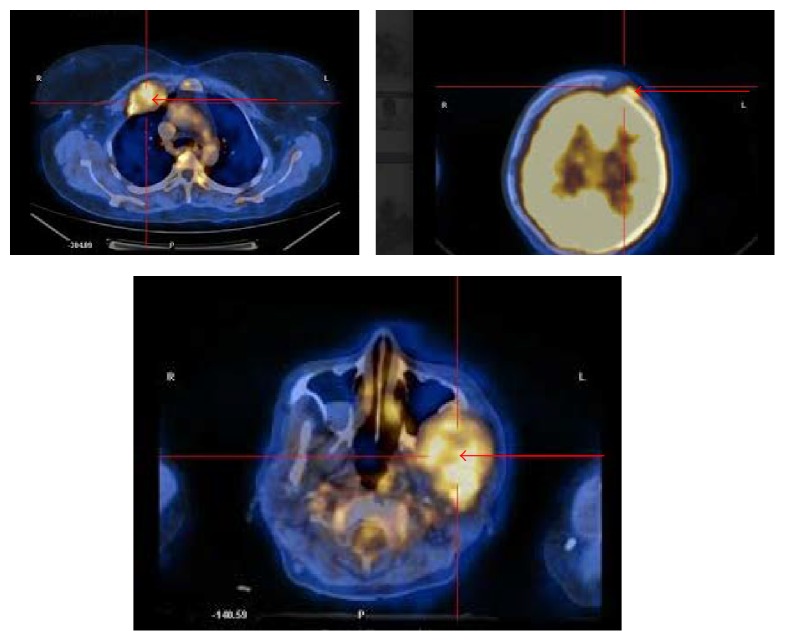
PET CT images showing extensive FDG uptake in frontal bone, maxilla, and right costochondral junction of second rib.
